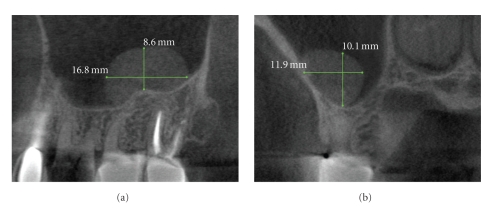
Antral mucosal pseudocysts, also called mucus retention cysts, are a relatively common localized dome-shaped antral mucosal swelling, often of allergic origin and while they can occur anywhere in the sinus present diagnostic challenges when associated with the floor of the maxillary sinus. CBCT imaging ((a) sagittal, (b) cross-sectional) can be useful in differentiating these lesions from periapical mucositis in that the former is usually not associated with disruption of the floor of the sinus and expansion superiorly from the apex of roots of adjacent teeth.
